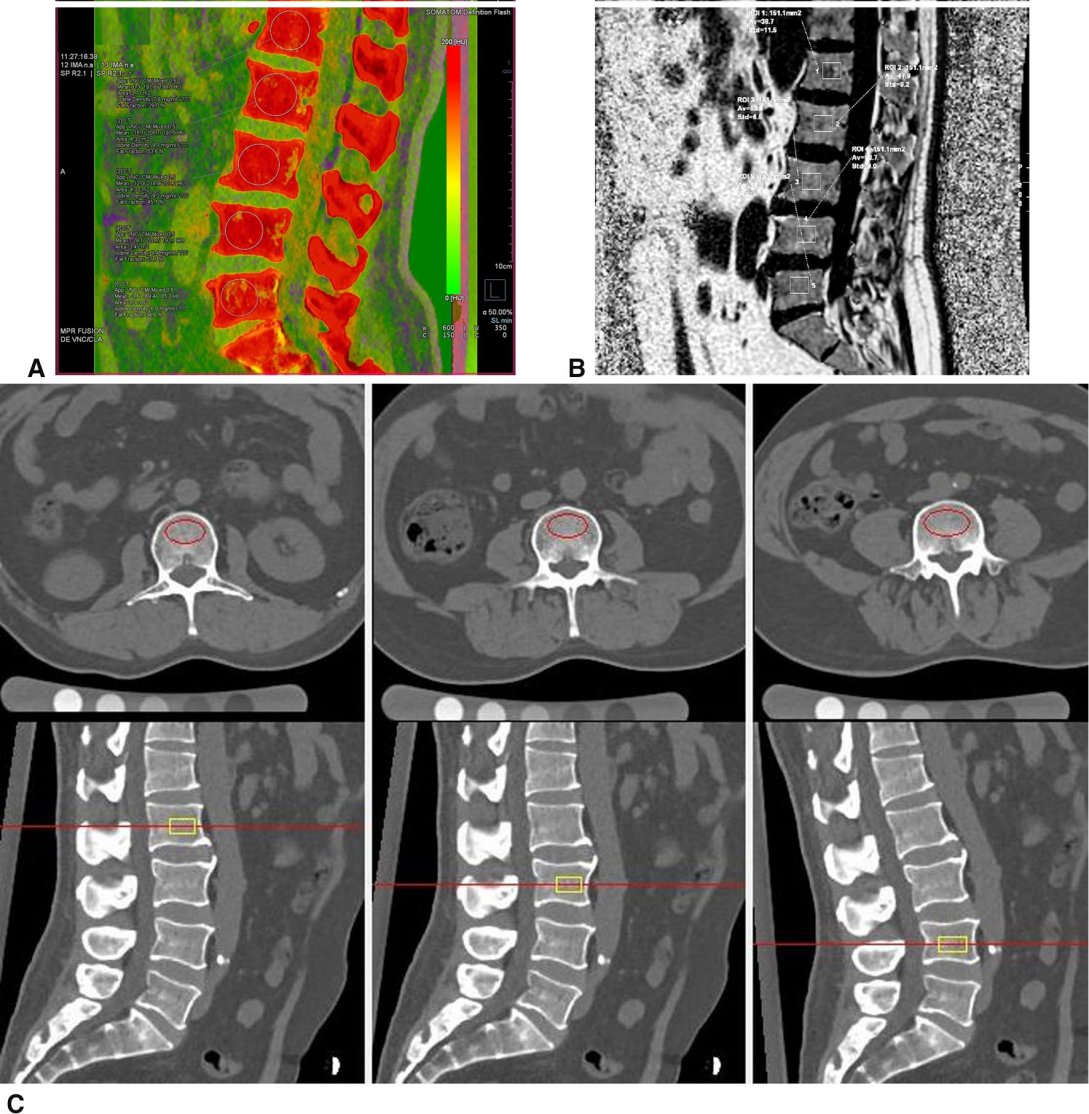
Measurement of DECT-FF, MRI-FF, and BMD: A, B DECT-FF and MRI-FF derived from corresponding region of interest (ROI) in a standard median sagittal plane, respectively, and the ROI was delineated in 2/3 of the anterior vertebral body, avoiding the bone cortex, vertebral vein sulcus, and surrounding osteosclerosis; C BMD determined by ROI automatically drawn with QCT analytics system
